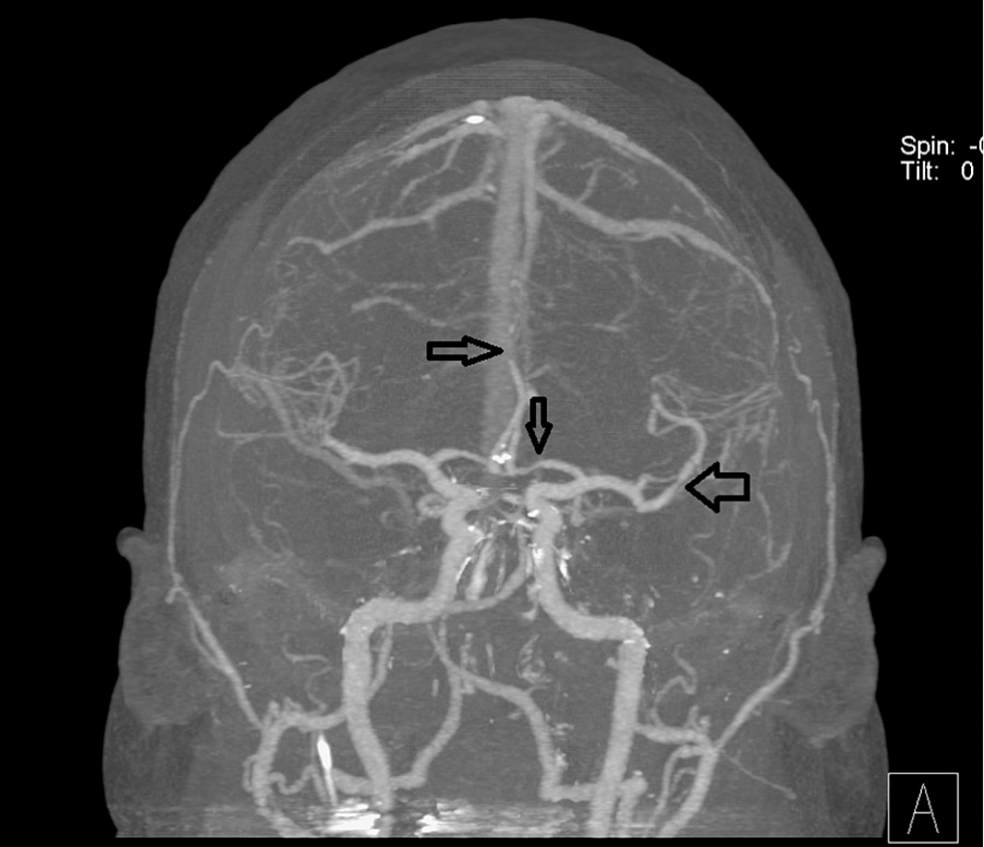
Initial computed tomography angiography (CTA) of the brain CTA showing multifocal stenosis affecting left M2, A1, and bilateral A3 (arrows).
